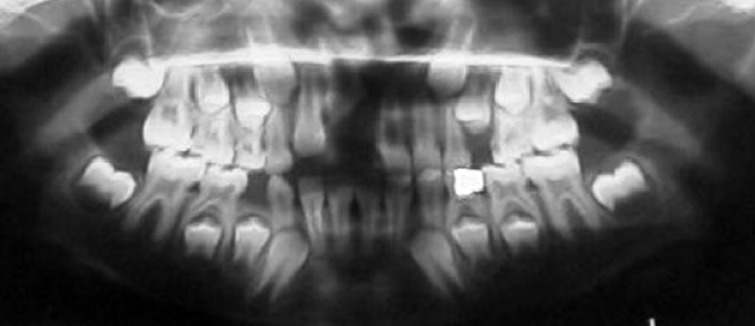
Panoramic radiography shows position of both intruded and extruded centrals in the maxilla
